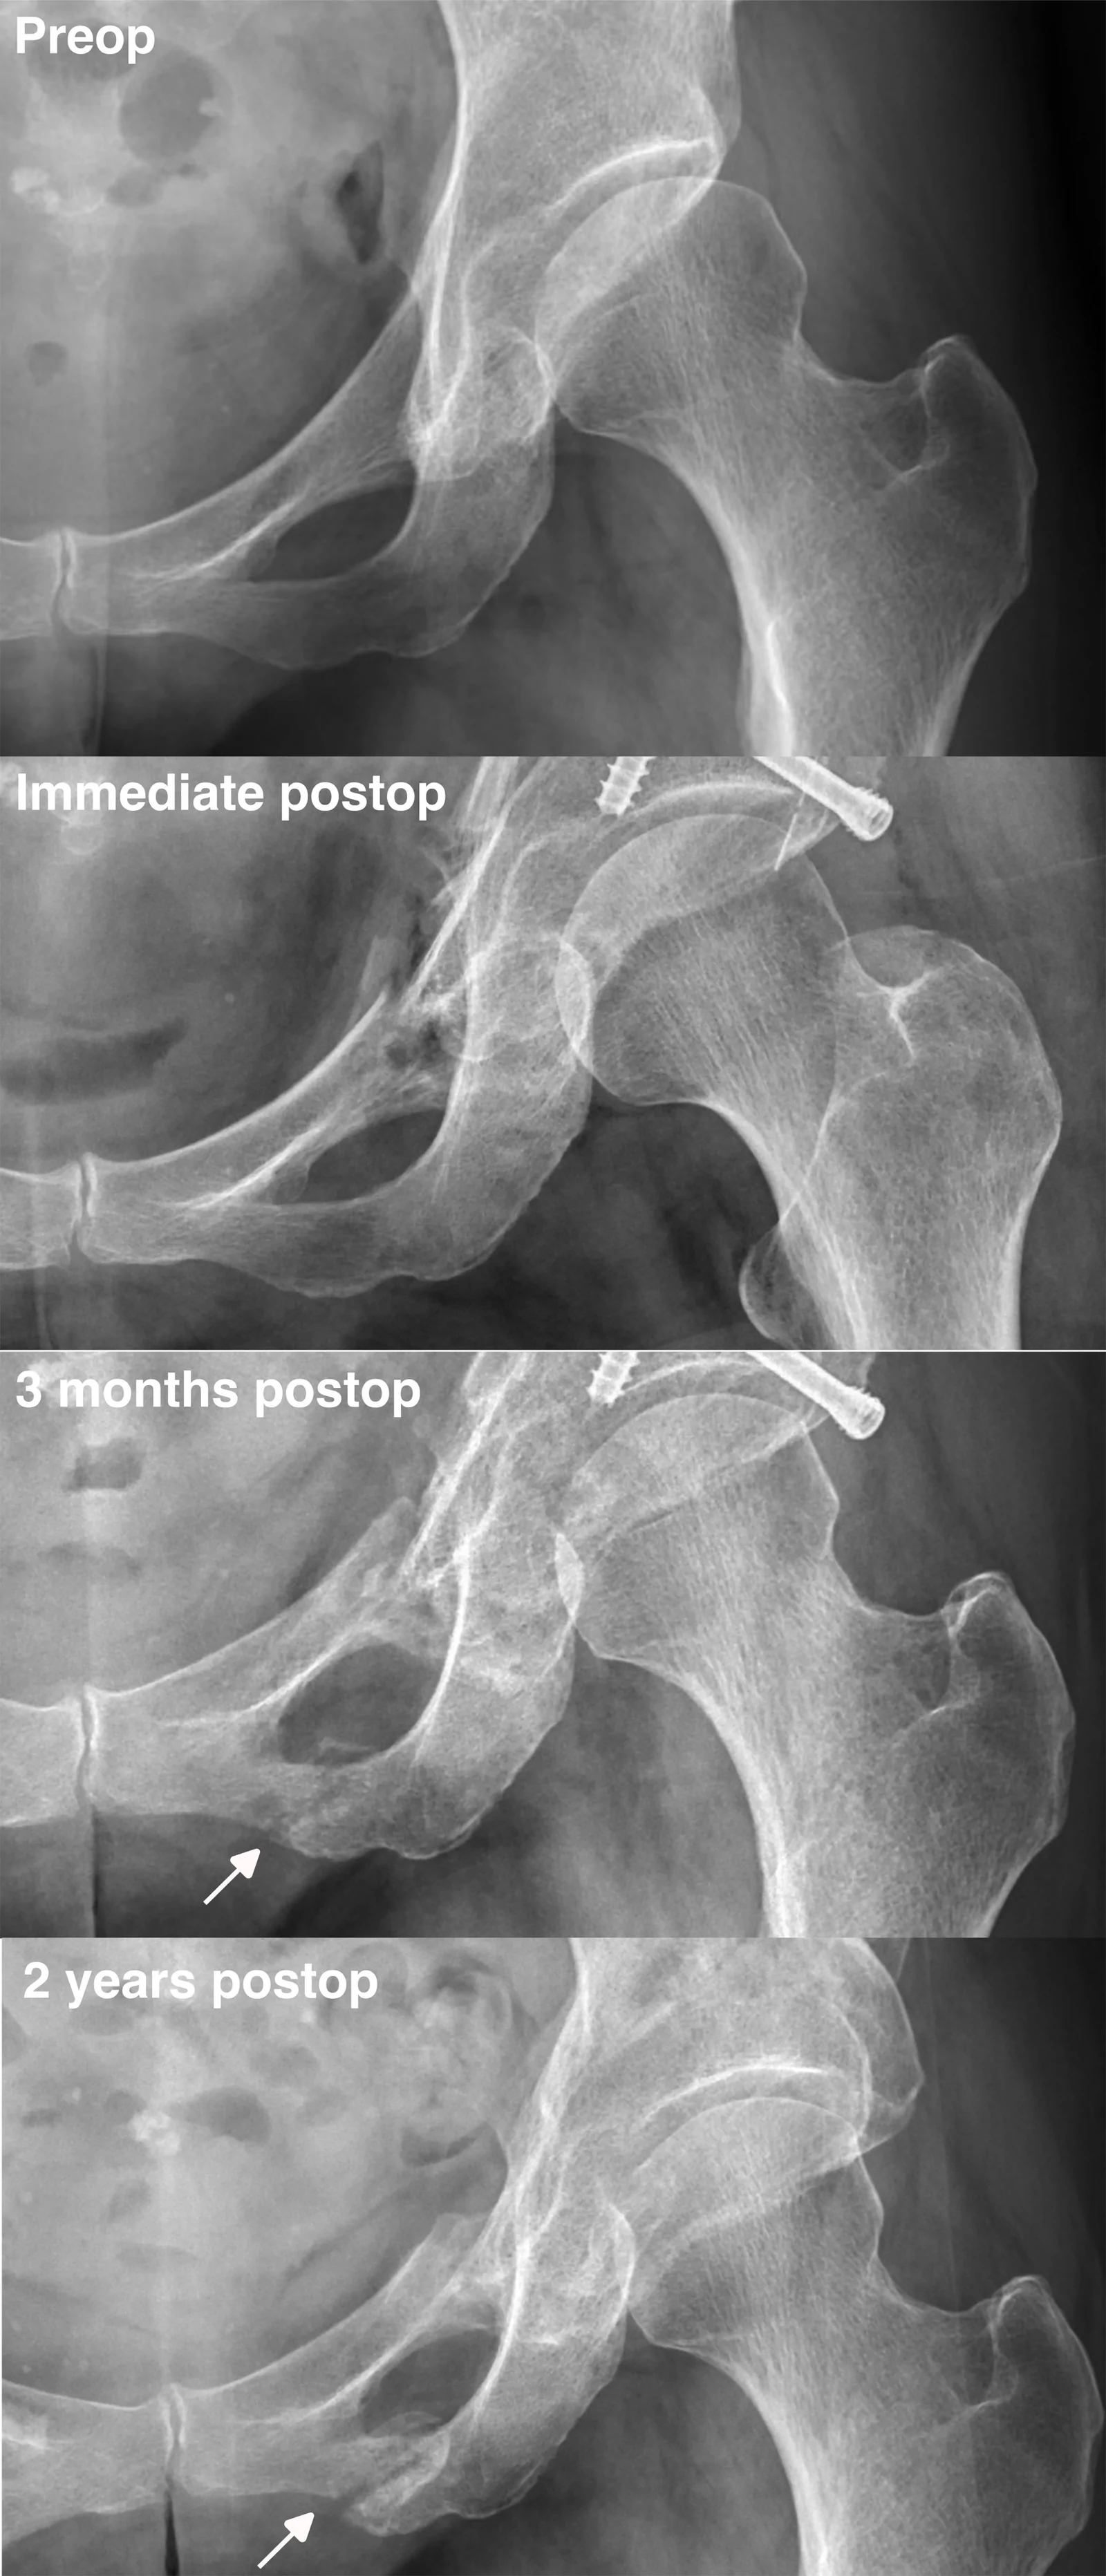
Serial radiological images of a 53-year-old female who underwent left PAO for symptomatic hip dysplasia. A left inferior pubic ramus stress fracture was first identified three months postoperatively (white arrow), with progressive surrounding callus formation observed on the two-year postoperative radiograph.
